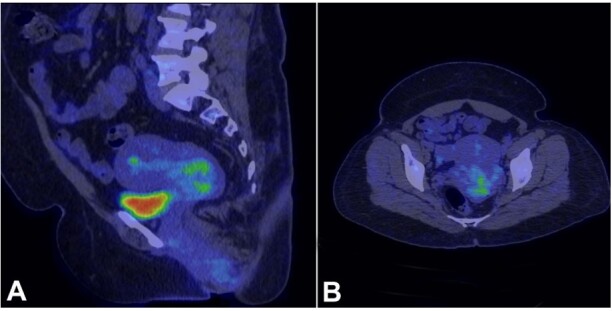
PET CT of the patient shows in A (sagittal) and B (axial) - a well-defined, slightly lobulated non-FDG avid lesion within the endocervical canal involving the posterior lip of the cervix and extending into the posterior cervical myometrium measuring 3.9x4x3.3cm, without invading into the uterus, urinary bladder, and the surrounding lymph nodes.
